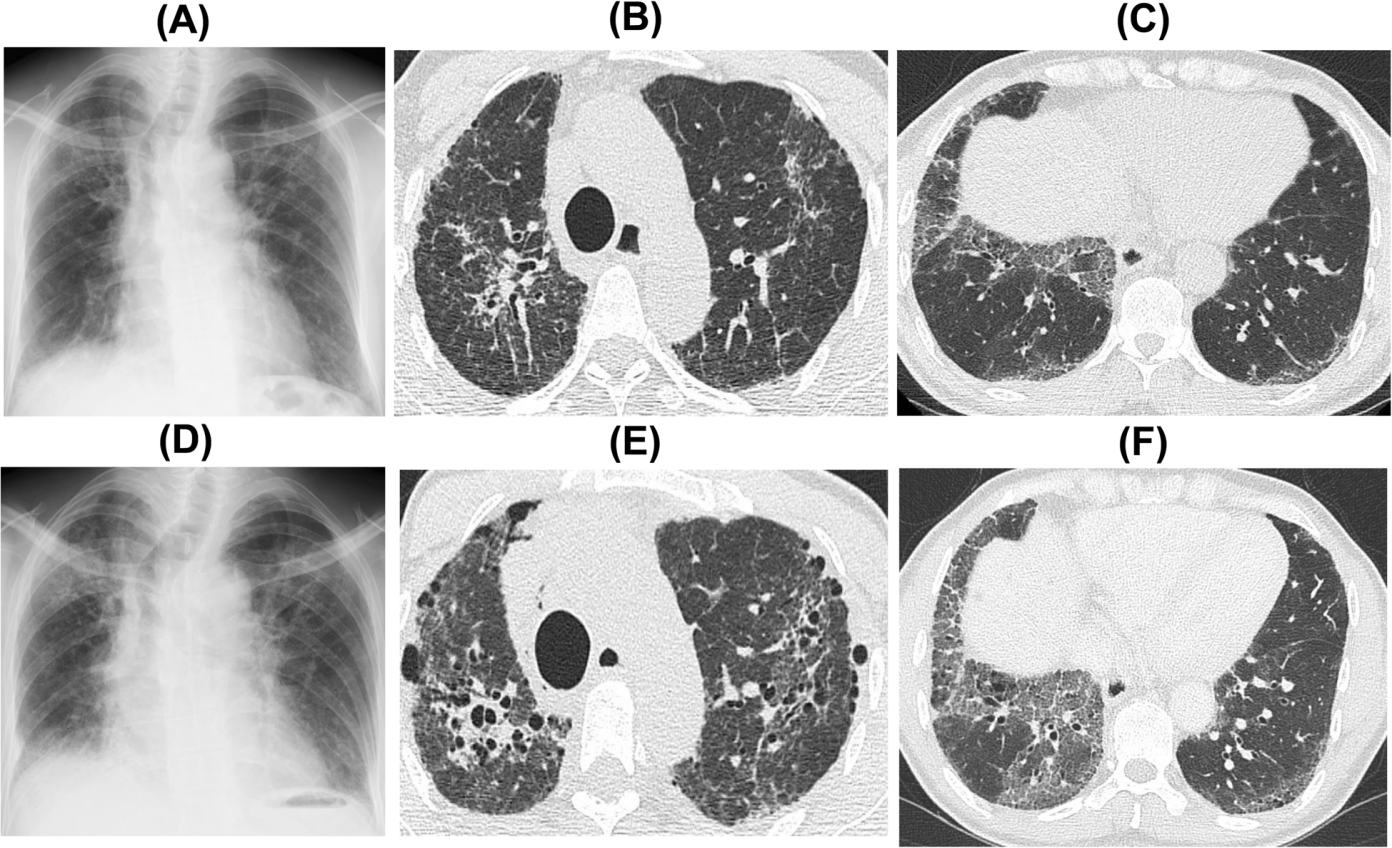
a Chest X-ray at the first visit of the patient to our hospital. Chest high-resolution computed tomography (HRCT) findings in the b upper and c lower lung fields at the first visit to our hospital. d Chest X-ray 2 years after the first visit to our hospital. Chest HRCT findings in the e upper and f lower lung fields 2 years after the first visit to our hospital
